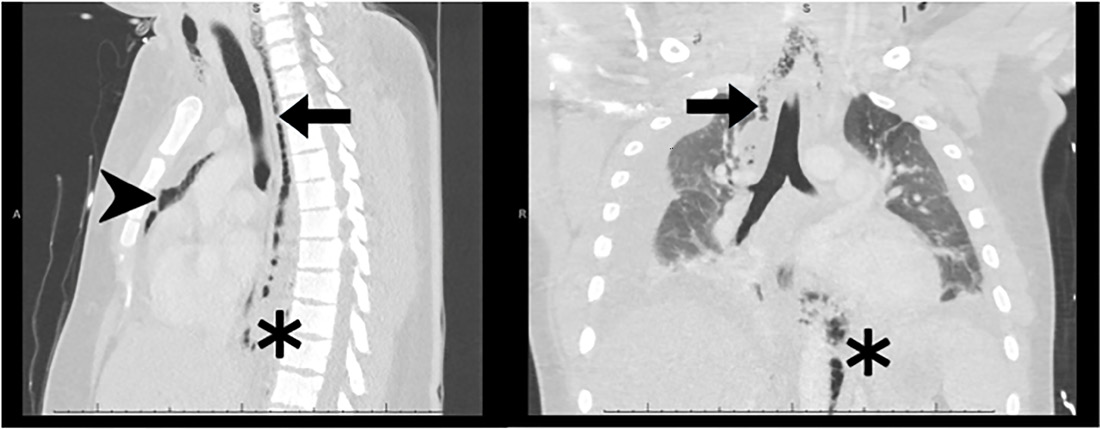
Computed tomography of the chest with intravenous contrast in coronal (right)
